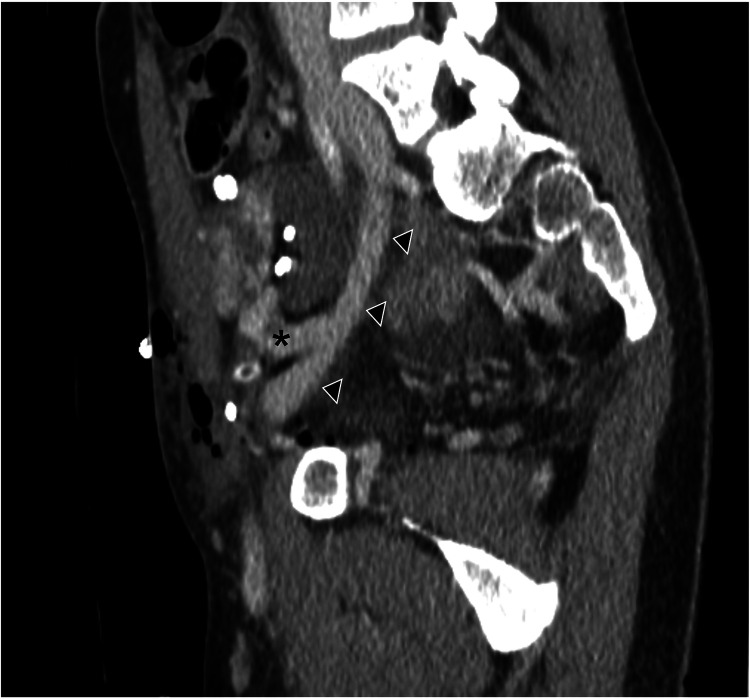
Postoperative recipient CT scan (multiplanar sagittal-oblique reconstruction related to case #7 in Table 1). Arrowheads indicate the course of the right external iliac vein; the asterisk indicates the cryopreserved vena cava patch. On postoperative day 2, the recipient underwent a CT scan for hypotension.
